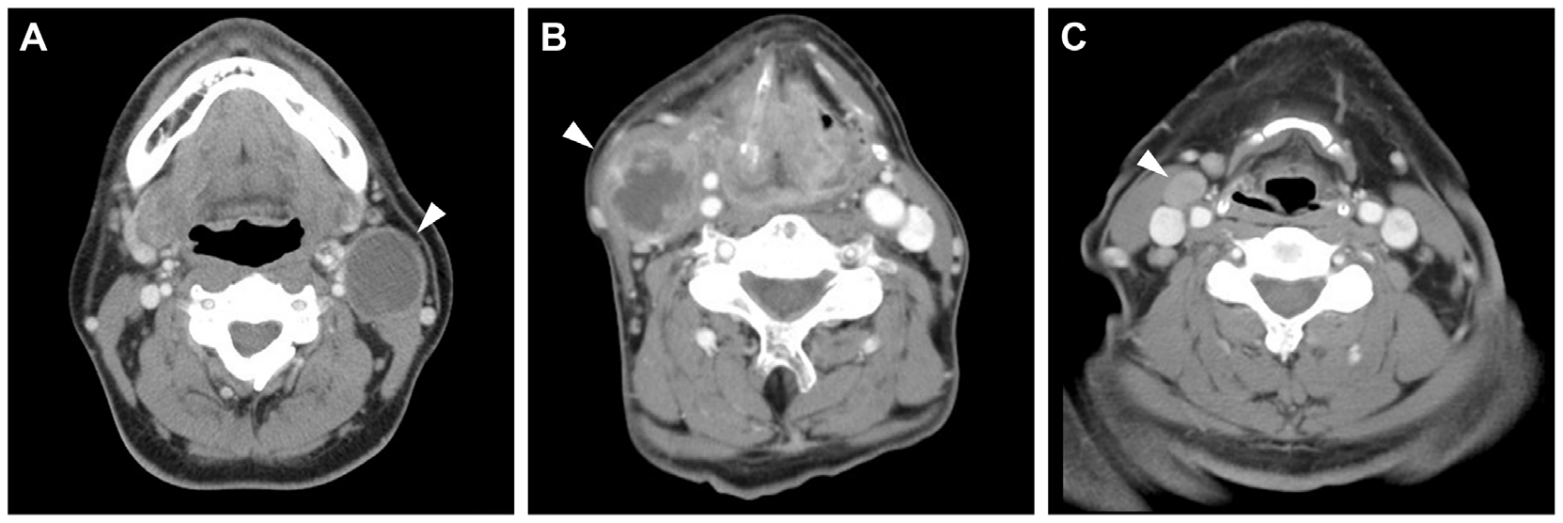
Radiographically identifiable (A) cystic, (B) necrotic, and (C) solid node metastasis defined by axial contrast-enhanced CT scans. Note a contrast-enhancing thin wall and homogeneous low-density content in the cystic node metastasis.
